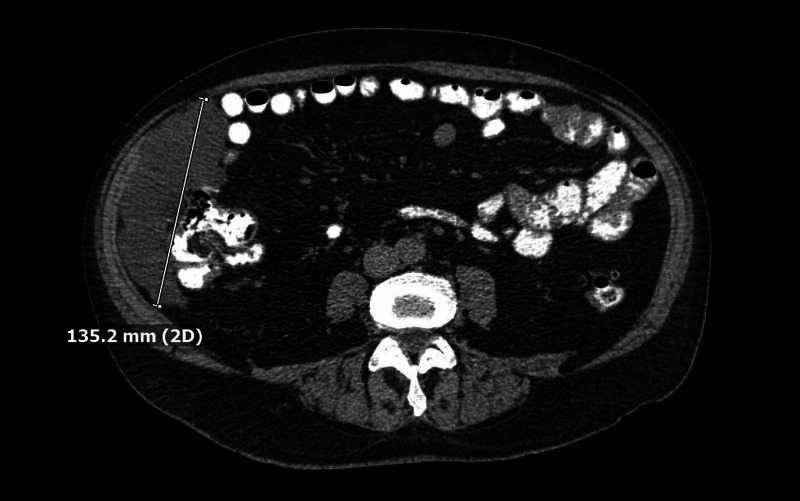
Abdominal CT (November 2018). CT of the abdomen showing a 13.5 cm right lower quadrant (RLQ) paracolic gutter fluid collection.
